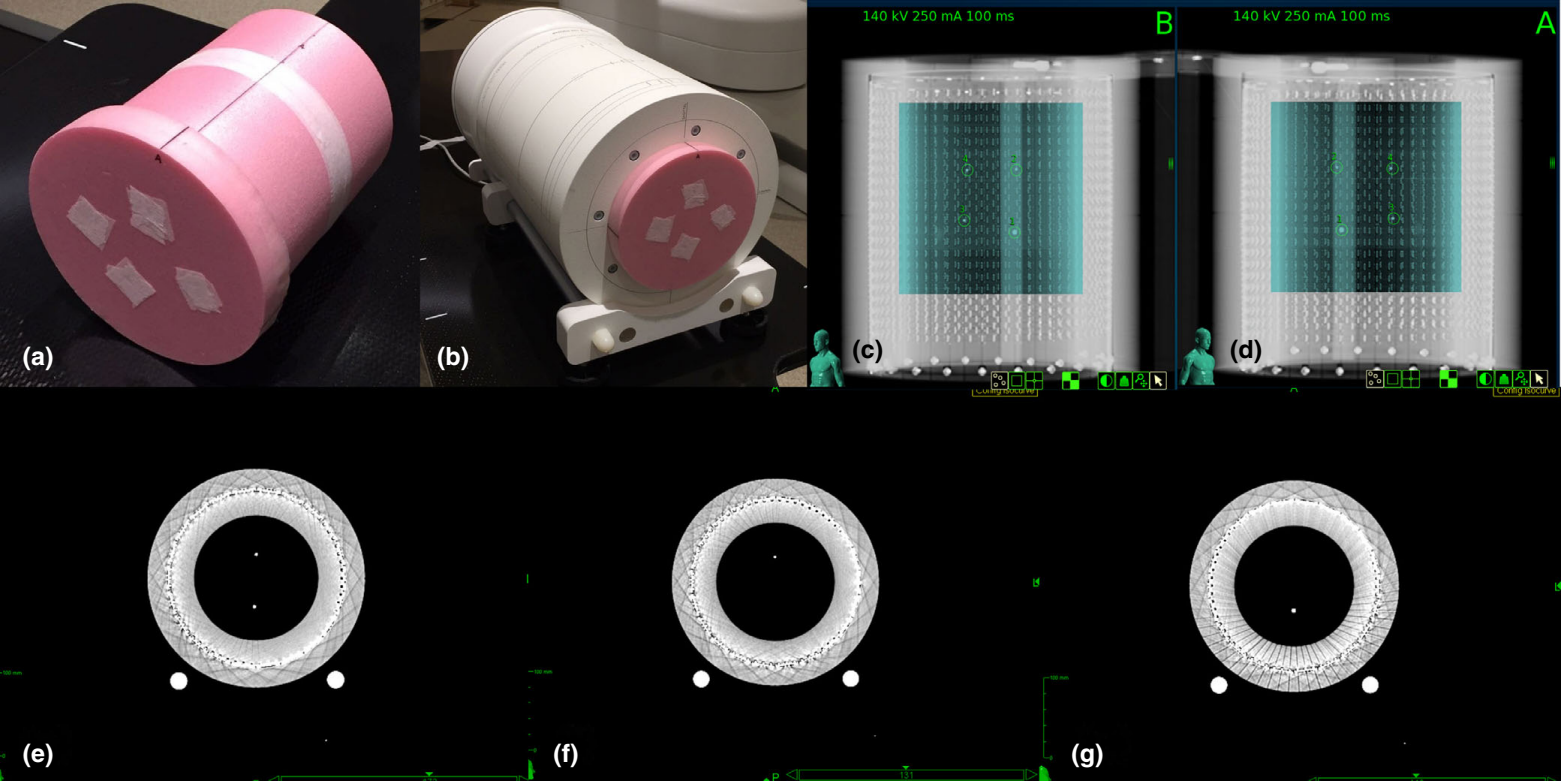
Photos of (a) tailor‐made Styrofoam with four implanted fiducial markers, (b) ArcCHECK device with the Styrofoam insert, (c), (d) Setup images of the ArcCHECK, (e)–(g) computed tomography images of the ArcCHECK with fiducials.
